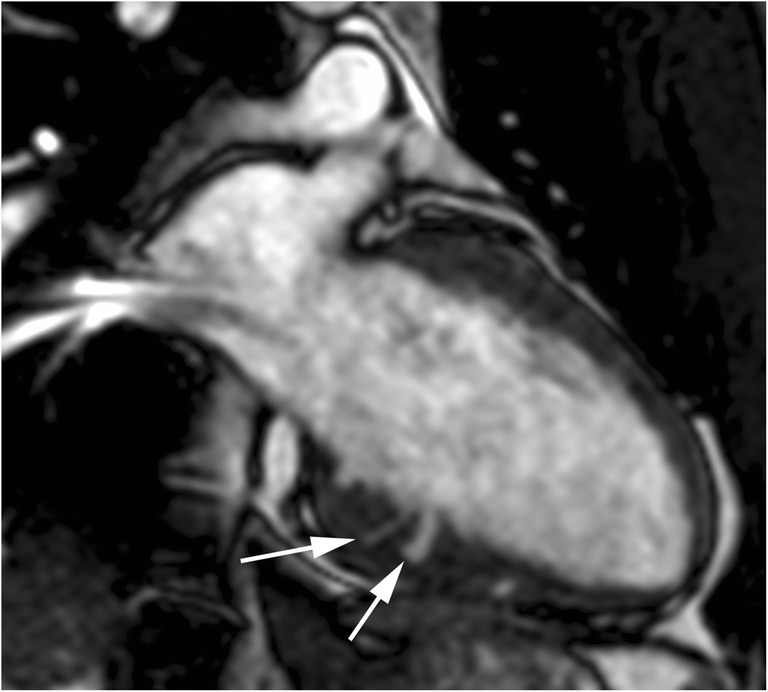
22-year-old man with myocardial crypts and a family history of HCM without myocardial hypertrophy. Two-chamber steady-state free precession (SSFP) cine MR image at end-diastole shows V-shaped myocardial crypts perpendicular to the endocardial border at inferior LV wall (arrows). The crypts close completely during systole (not shown)
